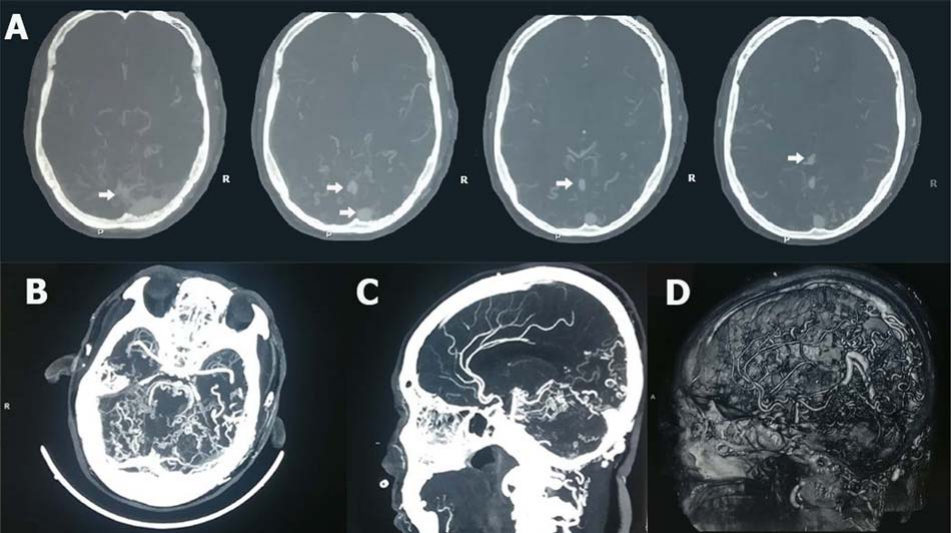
CTA of the head. CT cerebral angiogram (axial sections) in bone window settings (A), MIP in axial sections (B) and sagittal sections (C) and Volume Rendered Images in sagittal section (D) show multiple small-sized nidus (<3 cm) [shown by white arrows in (A)] fed by multiple normal to moderately enlarged feeder arteries with absence of early venous drainage, predominantly involving the bilateral posterior cerebral artery, superior cerebellar artery, anterior inferior cerebellar artery and posterior inferior cerebellar artery territories.
